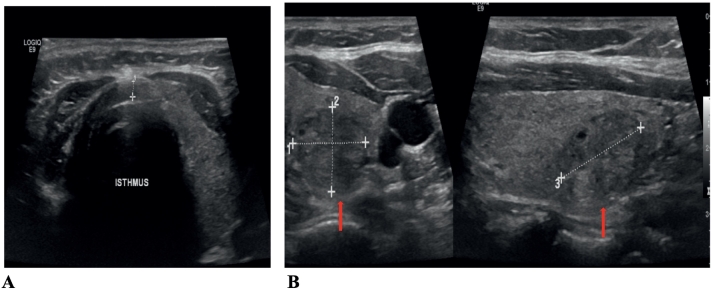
(A) Transverse ultrasound view of the neck at the thyroid gland level showing absent right thyroid lobe tissue; (B) transverse and longitudinal view of left lobe of thyroid gland showing solid heterogenous slightly hypoechoic nodule (red arrow).
